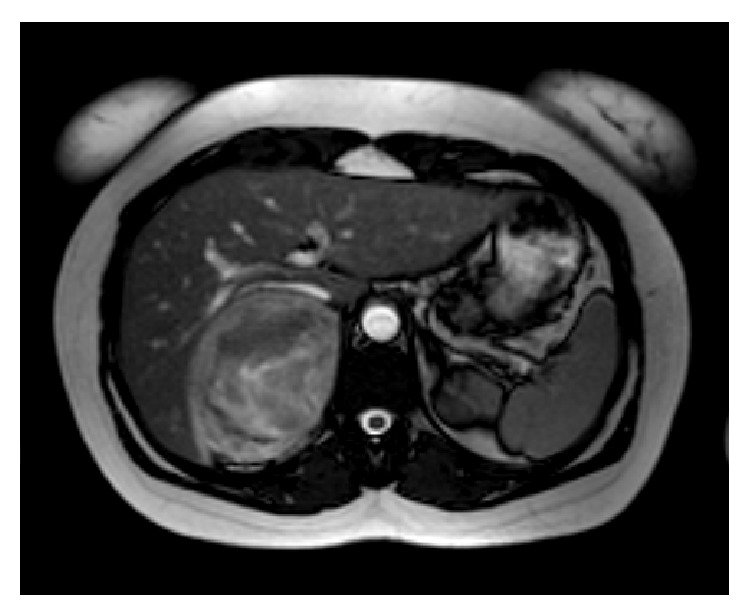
MRI describing mass of Dmax = 9 cm between right kidney and liver, with hemorrhagic elements.
